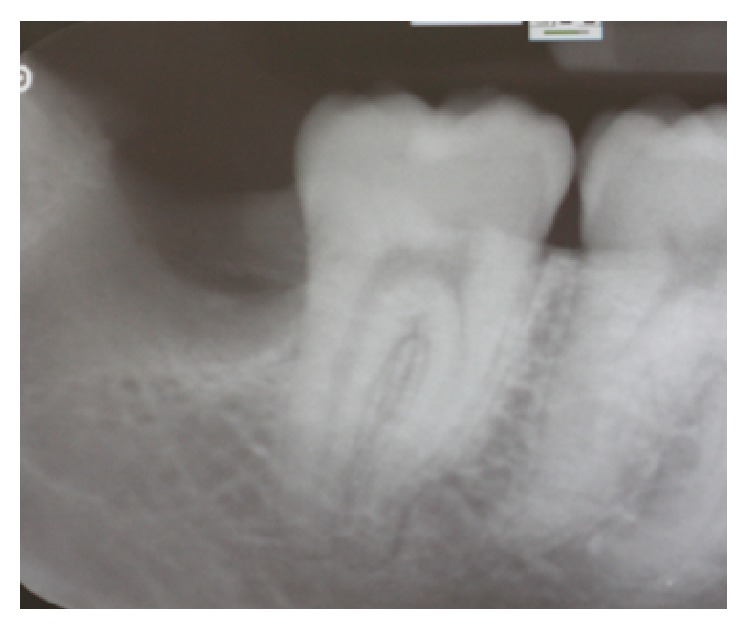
Preoperative periapical X-ray of the lower right second molar.
